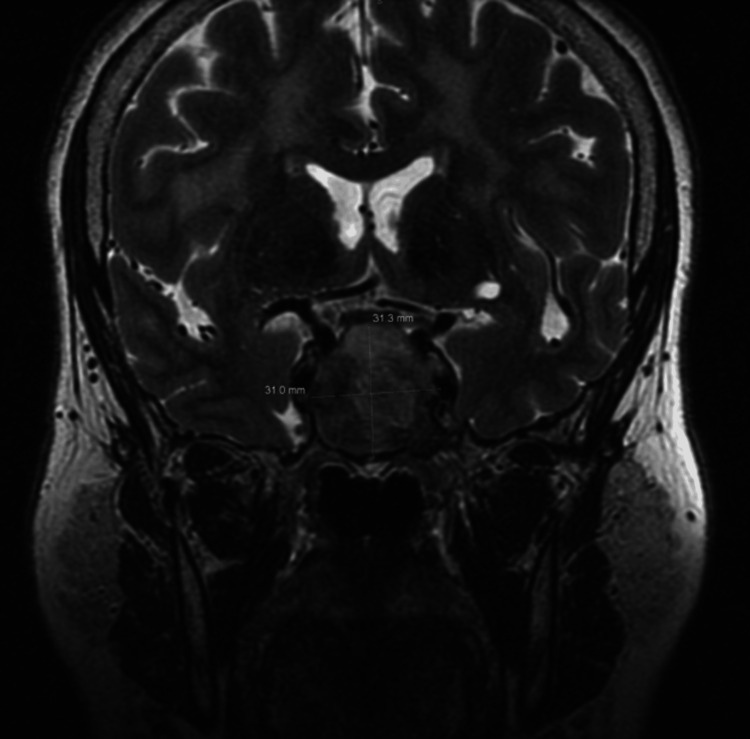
MRI findings portraying a large pituitary macroadenoma measuring approximately 4 cm anteroposteriorly, 3.1 cm side to side, and 3.1 cm cephalocaudally, with extension into the sphenoid sinus MRI: magnetic resonance imaging
